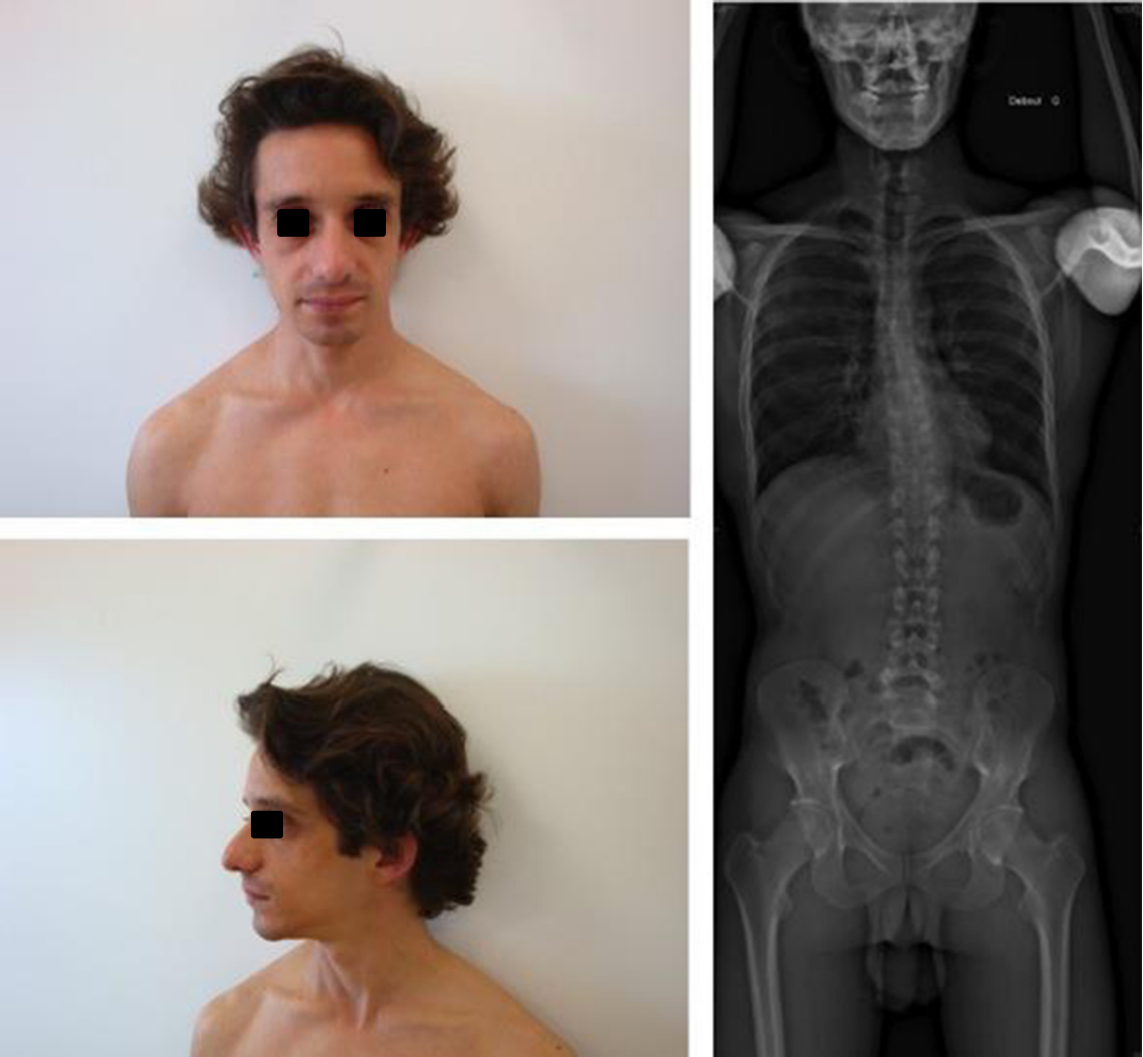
Facial dysmorphism of patient (a face and b profile) and X-Rays showing the scoliosis (c).
